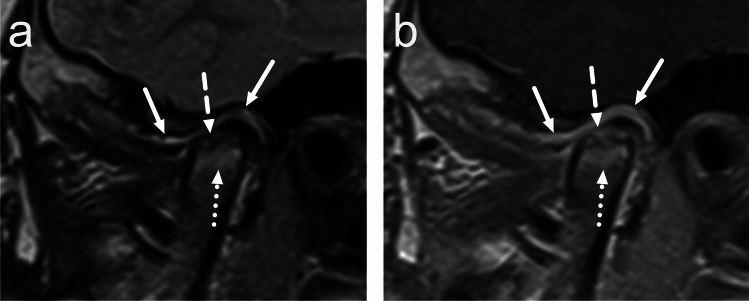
Oblique T2-weighted (a) and contrast-enhanced T1-weighted (b) MR images of the left TMJ of a 4-year-old female showing synovitis (arrows), bone marrow oedema/enhancement (dotted arrows), and condylar irregularities/erosions (dashed arrows)
